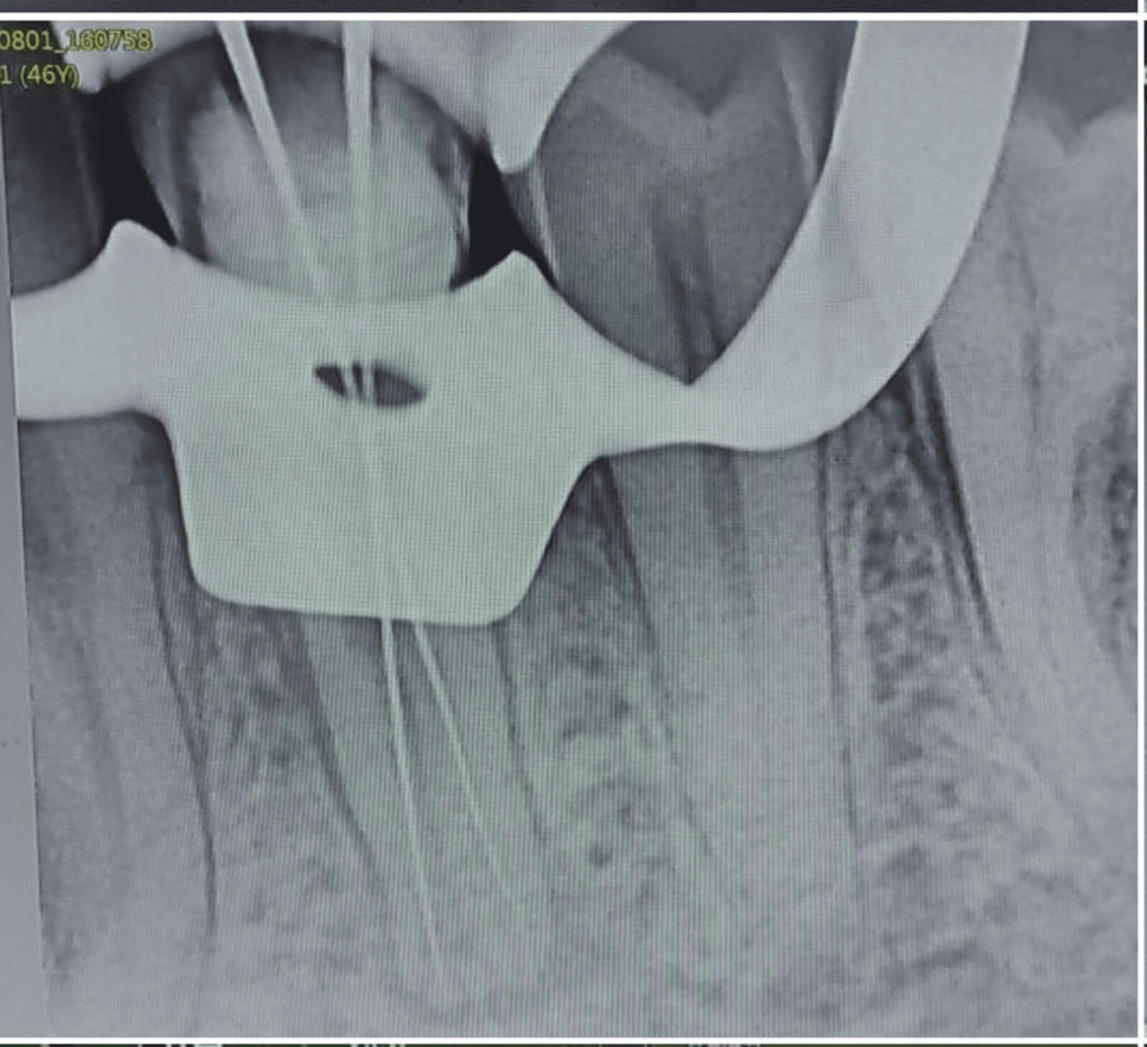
The patency of both canals was confirmed with the #10 K file (Mani Inc., Tochigi, Japan). Subsequently, the initial working length was determined using the #15 K file (Mani Inc., Tochigi, Japan) with the help of Root Zx Mini Apex Locator (J. Morita Corp., USA), which was evaluated using a radiograph
